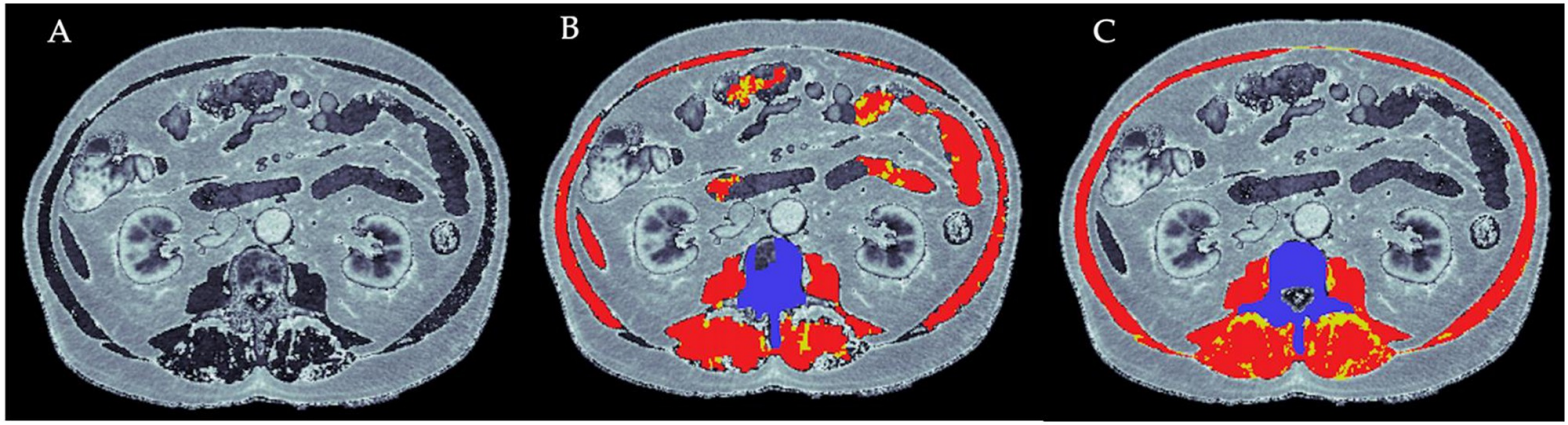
Assessment of total L3 level muscle mass and intramuscular fat. A) Raw L3-level CT central slice (0.82 x 0.82 x 2.5 mm) depicting muscle in dark grey (organs are also shown in dark grey) and fat in light grey. B) L3-level muscle, intramuscular fat, and bone segmentations achieved using the fully-automated algorithm. C) L3-level muscle, intramuscular fat, and bone segmentations after manual correction of automated outputs from B, using Slice-O-Matic software. Skeletal muscle segmentations are shown in red, fat in yellow, and bone in purple. Note: Processing of the image using the automated algorithm (B) resulted in mislabeling of some organs as muscle or fat. These inaccuracies were corrected using Slice-O-Matic (TomoVision, Montreal, Canada) (C).
